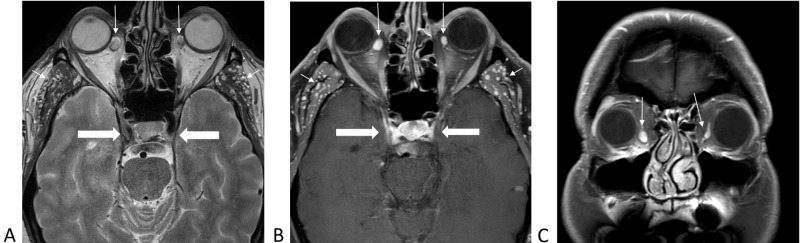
Magnetic resonance imaging of the brain demonstrating varices of inferior ophthalmic veins bilaterally (long arrows), infratemporal venous vessels (short arrows), and normal cavernous sinuses (thick arrows). 1A, axial T2-weighted without contrast. 1B, axial T1-weighted with contrast and fat saturation. 1C, coronal T1-weighted with contrast and fat saturation.
